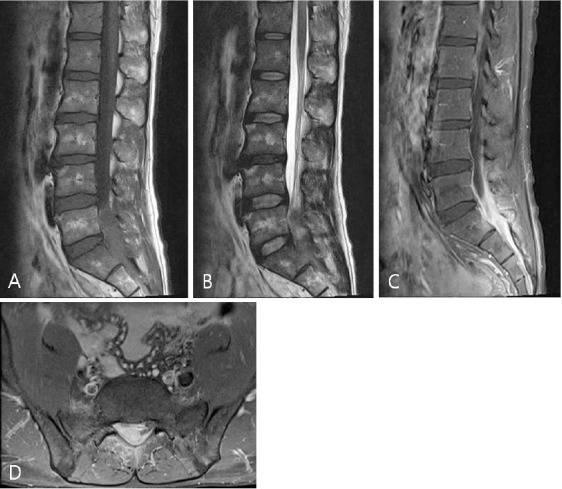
(A) MRI reveals an expansile right epidural mass from L4-5 to S2, showing homogeneous isointensity on the sagittal T1-weighted image, (B) heterogeneous iso- and hypointensity on the sagittal T2-weighted image, (C) sagittal gadolinium-enhanced T1-weighted image, and (D) axial image; a strong enhancing lesion with cord compression and abnormal signal intensity was detected in the adjacent back muscle
